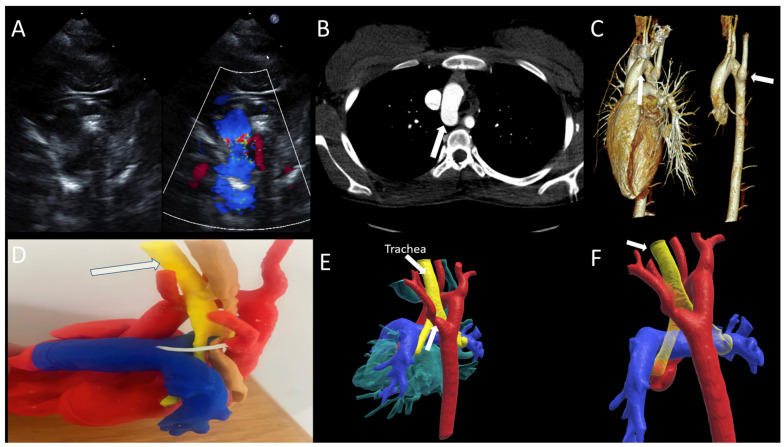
(A) Two-dimensional and Color Doppler TTE demonstrating RAA with aberrant left subclavian artery; (B,C) CT scan highlighting RAA from anterior and lateral views identifying the vessel contributing to airway compression; (D) 3D print; (E,F) VR reconstruction detailing the trachea in yellow and RAA.
